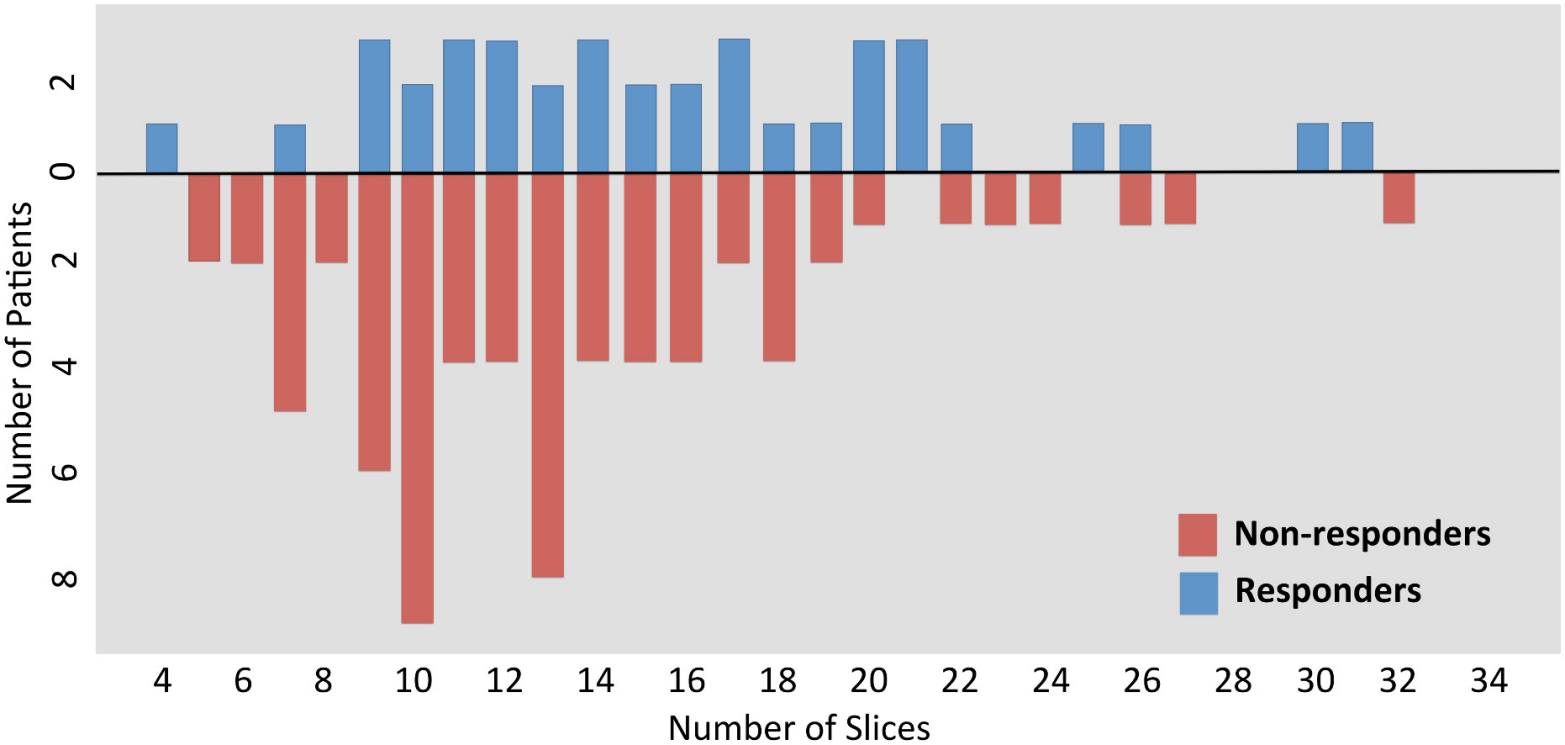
Distributions of axial ^18^F-FDG PET intra slices extracted from the 3D tumor volume of non-responders and responders.
